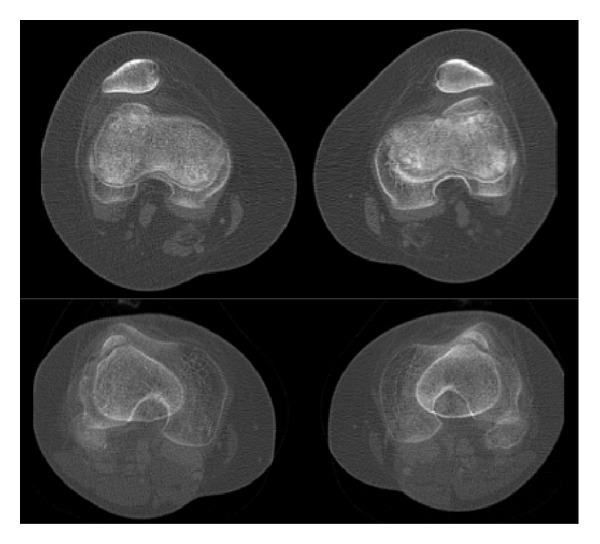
Top: CT-scan of both knees performed at the age of 15 years, showing bilateral patellofemoral dysplasia and permanent subluxation of patella. Bottom: distance TT-TG (tibial tubercle-trochlear groove) of 19 and 27 mm at the left and right side, respectively.
